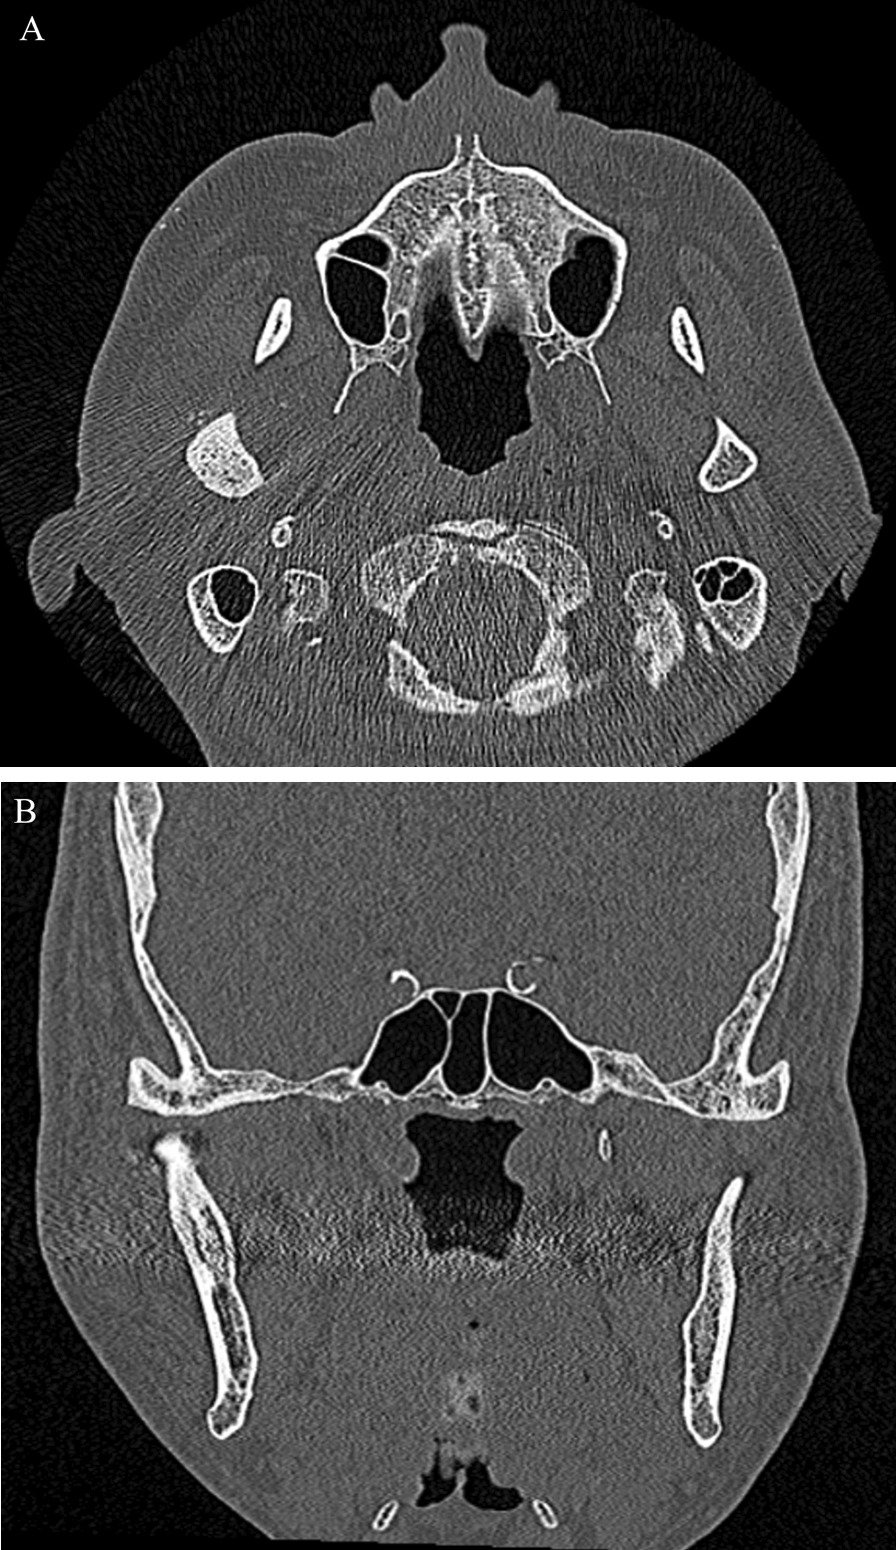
Postoperative CT images. A Axial CT images, and B coronal CT images confirmed the removal of the masses from the right TMJ
